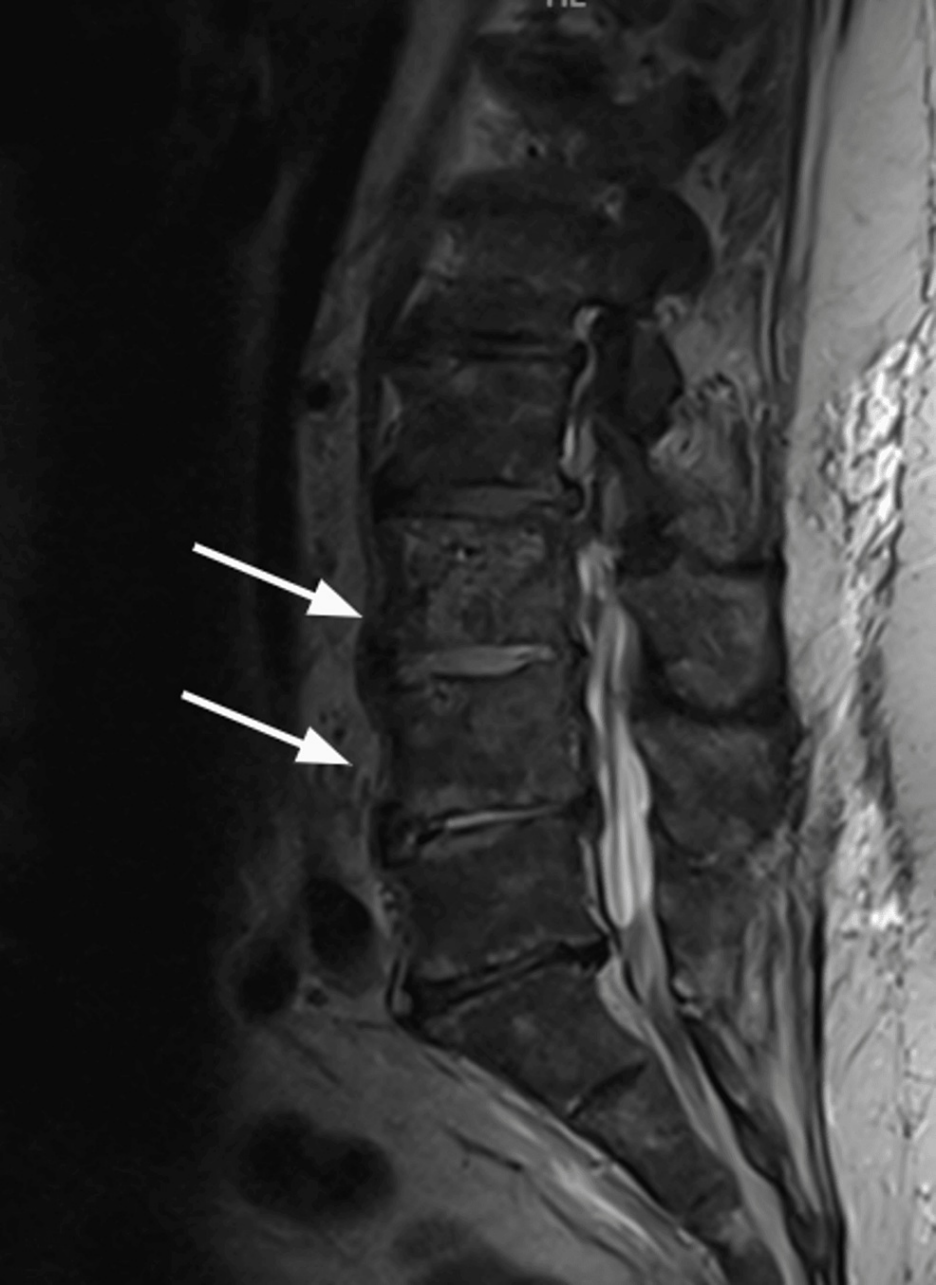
MRI SAG T2 of the lumbar spine showing moderate marrow edema in the L3 and L4 vertebrae with fluid in the L3-L4 and L4-L5 discs concerning for discitis/osteomyelitis.
